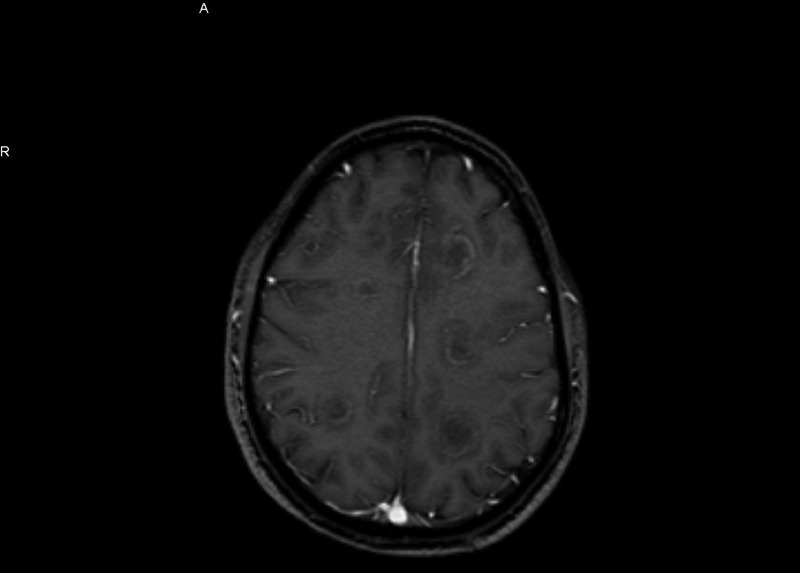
MRI without contrast shows ring-enhancing multiple bilateral lesions.
